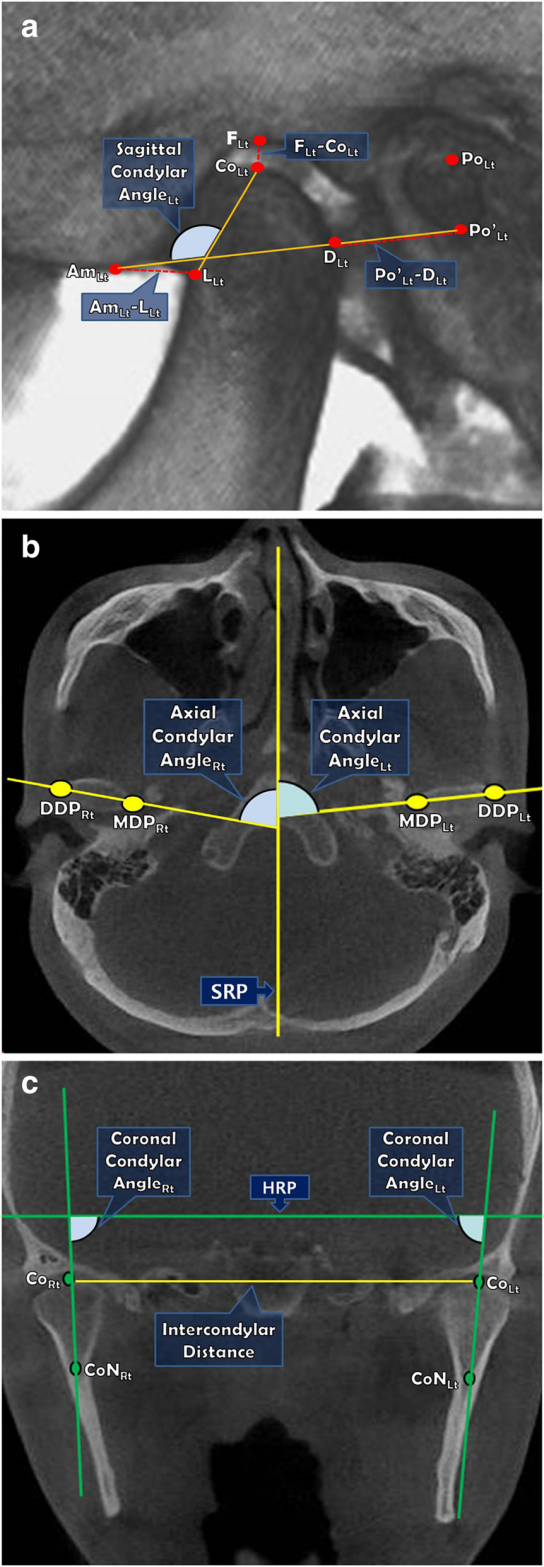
Measurements on 3D CBCT images.
